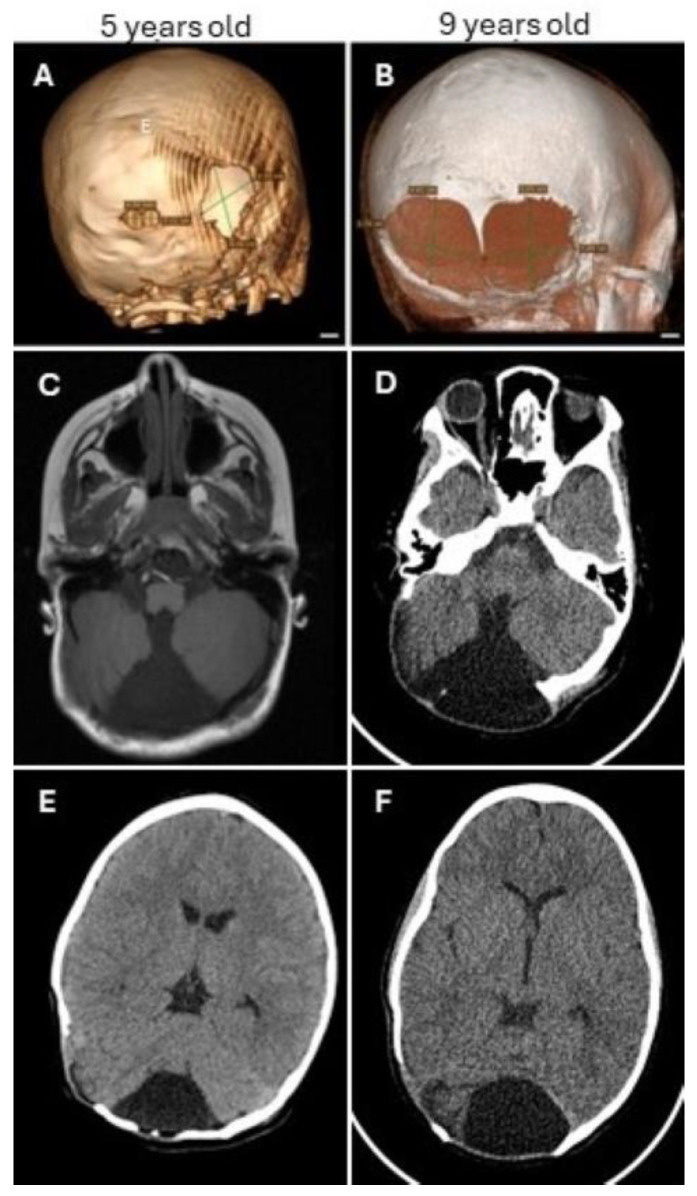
(A,B) Three-dimensional CT images demonstrating the progression of the bone defect for four years in the right occipital skull extending along and posterior to the lambdoid suture, at 5 years old (A) and 9 years old (B). (C,E) Images obtained at 5 years old: (C) axial T1 MRI demonstrating a large posterior fossa cyst displacing the cerebrum, and (E) axial CT image showing the bone defect in the right occipital skull along the lambdoid suture with an underlying mild bulge of the dura and brain parenchyma through the defects. A smaller defect is present in the posterior central part of the occipital bone. (D,F) Axial CT images obtained at 9 years old show the enlargement of both the defect and the cyst.
